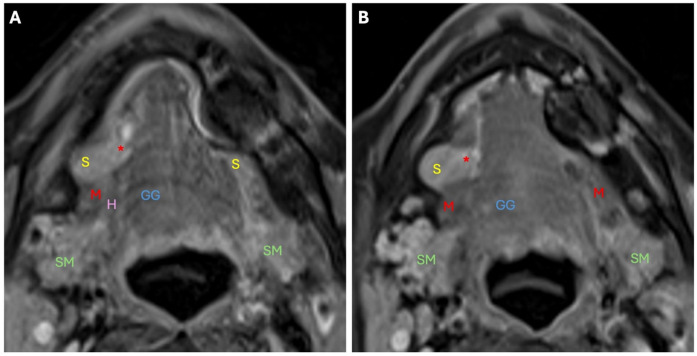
The two consecutive axial postcontrast T1-weighted fat-saturation images above (A) and below (B) show herniation of the right sublingual gland (S) through the right mylohyoid boutonniere (*) into the right submandibular space. The left sublingual gland is smaller in comparison. S: sublingual gland; SM: submandibular gland; M: mylohyoid muscle; *: Boutonniere; G: geniohyoid muscle; GG: genioglossus muscle; H: hyoglossus muscle.
